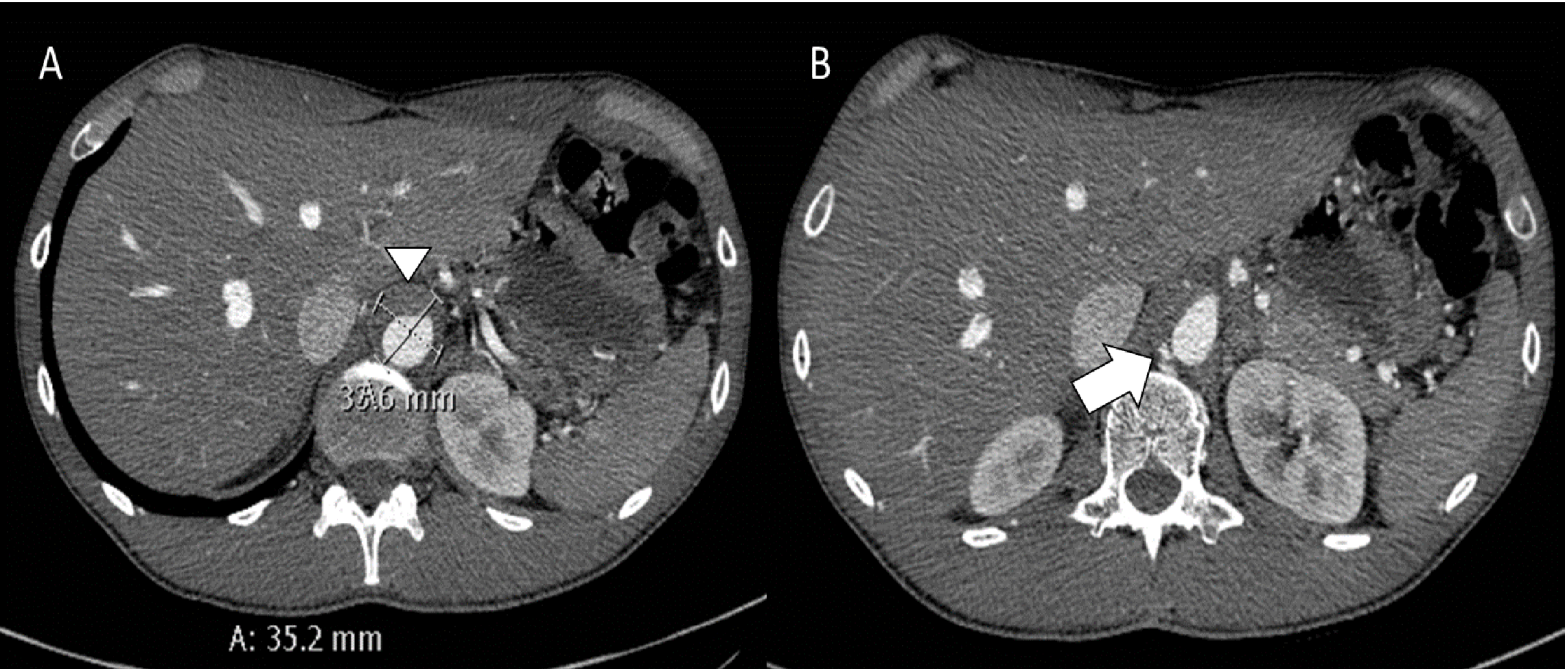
Axial contrast-enhanced computed tomography (CT) of the upper abdomen A) CT images showing an intramural hematoma with an aneurysm (arrowhead). B) Axial CT showing another small penetrating ulcer (white arrow).
